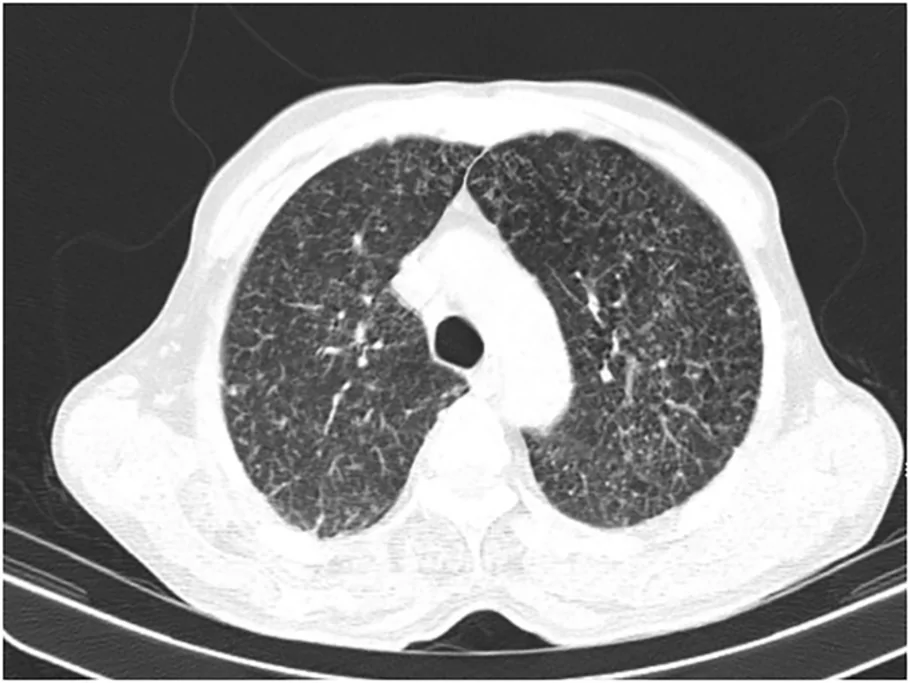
High‐resolution computed tomography (HRCT) of the chest demonstrating multiple diffuse bilateral micronodules, consistent with disseminated pulmonary tuberculosis.
